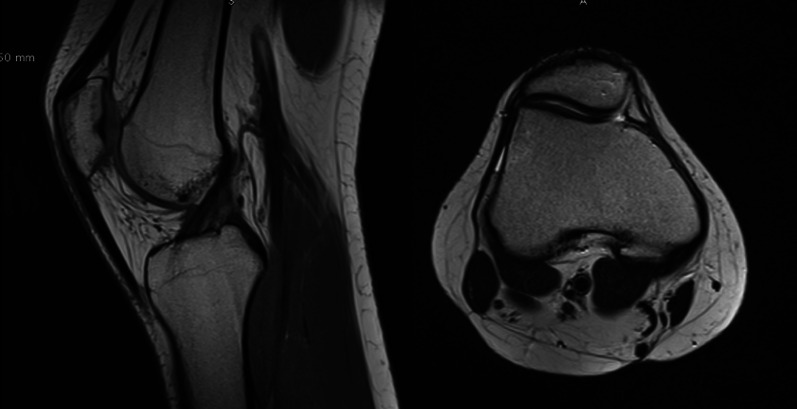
Right knee; MRI sagittal and axial view; patella alta (Insall–Salvati ratio 1.9) in modest external tilt in the presence of dysplasia by the shallow femoral trochlea
